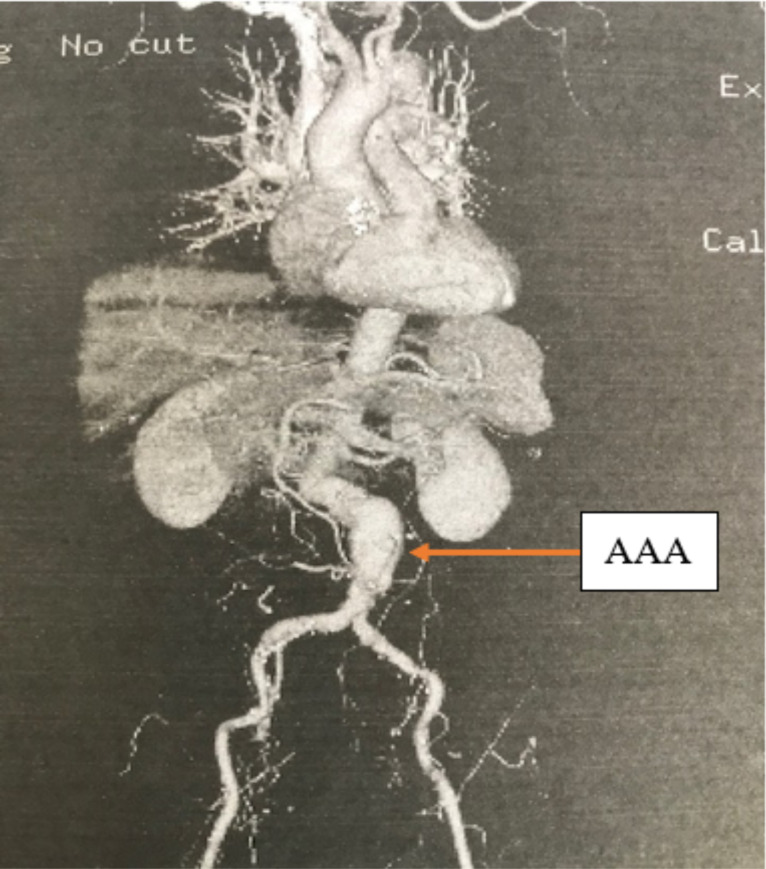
Pre-operative CT Angiogram
